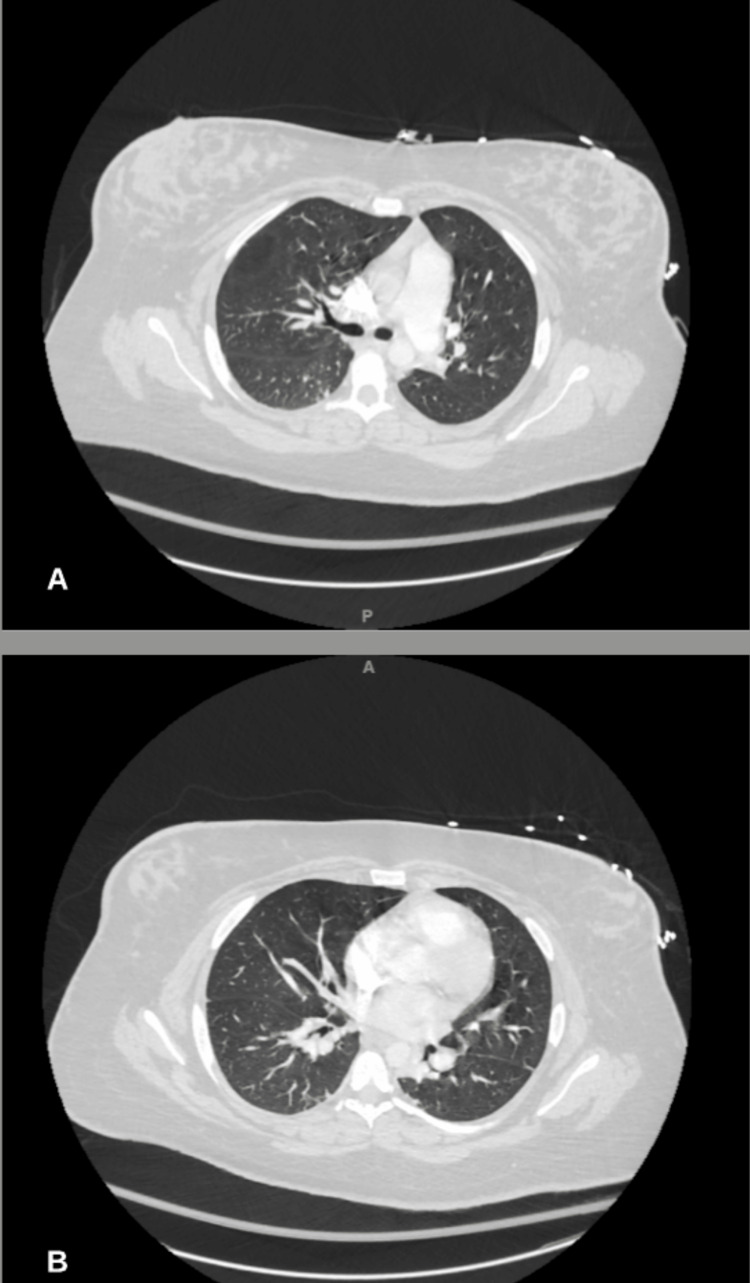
CTA unremarkable for pulmonary embolism or any other acute cardiopulmonary processes; A: Normal lower lung fields; B: Normal upper lung fields. CTA: computed tomography angiography.
